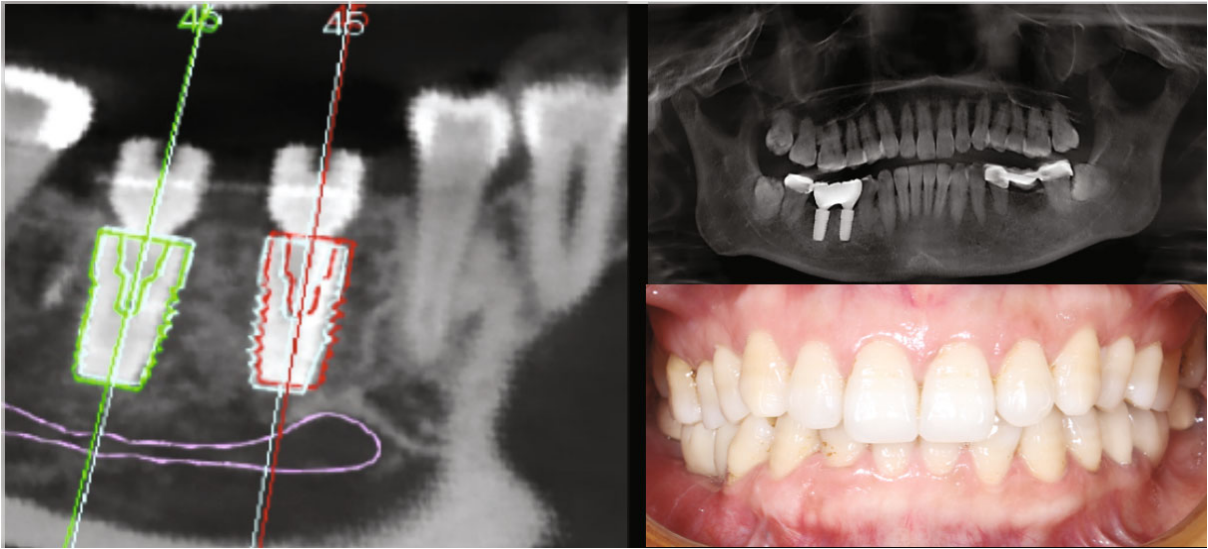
Differences between the intended (planned) and actual (postsurgery) positions of the implants and the final posttreatment radiograph and clinical image of the definitive restoration.
